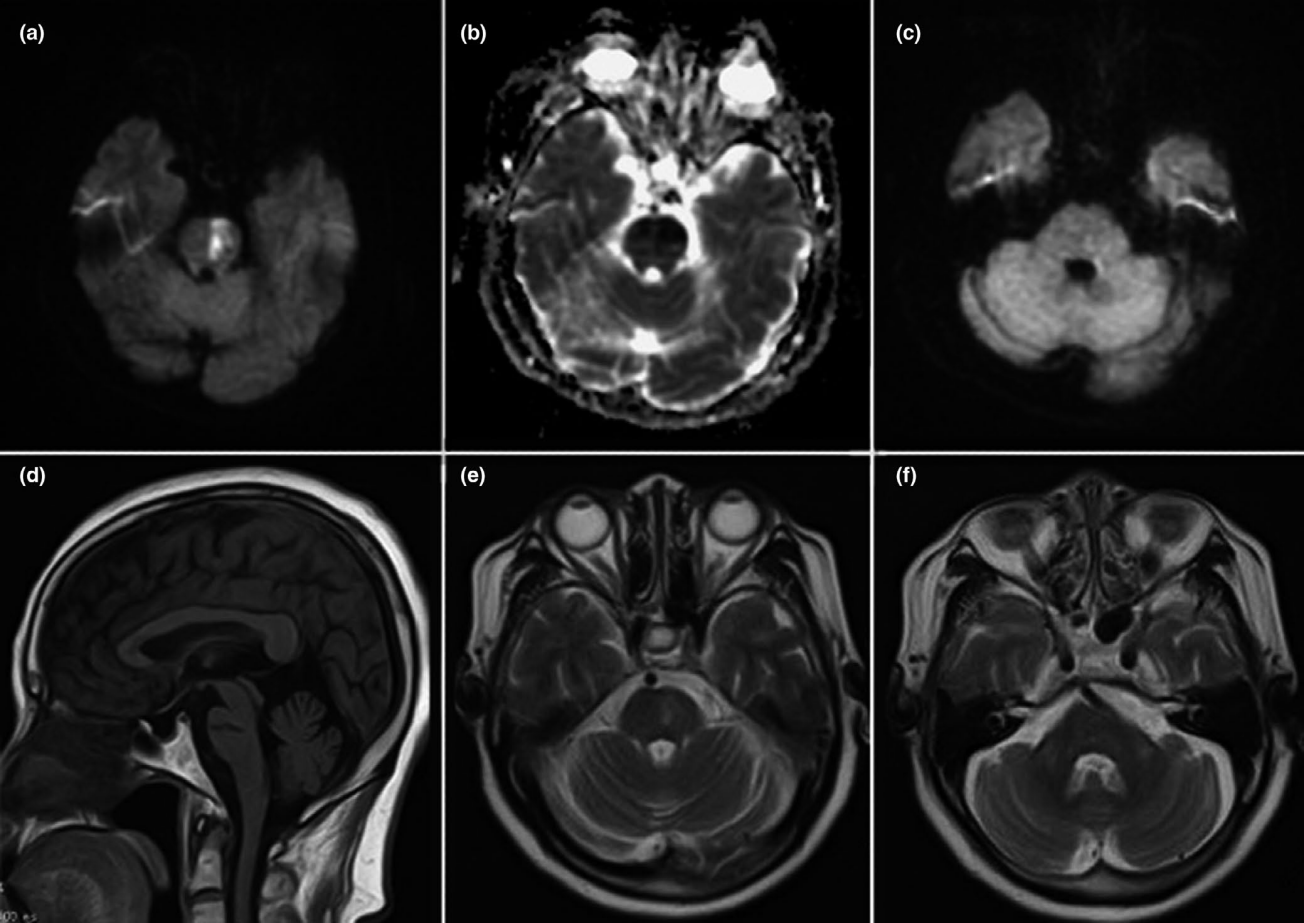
A 69‐year‐old woman with Wallerian degeneration after pontine infarction. Initial diffusion‐weighted imaging shows restricted diffusion of the left pons (a), with corresponding hypointensity on the apparent diffusion coefficient map (b) consistent with acute infarction. At this time, no MCP lesion is visible (c). On follow‐up MRI 4 months later, hypointensity on sagittal T1‐weighted imaging (d) and hyperintensity on pontine T2‐weighted imaging (e) indicates a previous infarction. Symmetrical hyperintensity of both MCPs on T2‐weighted imaging (f) suggests Wallerian degeneration of the pontocerebellar fibers
